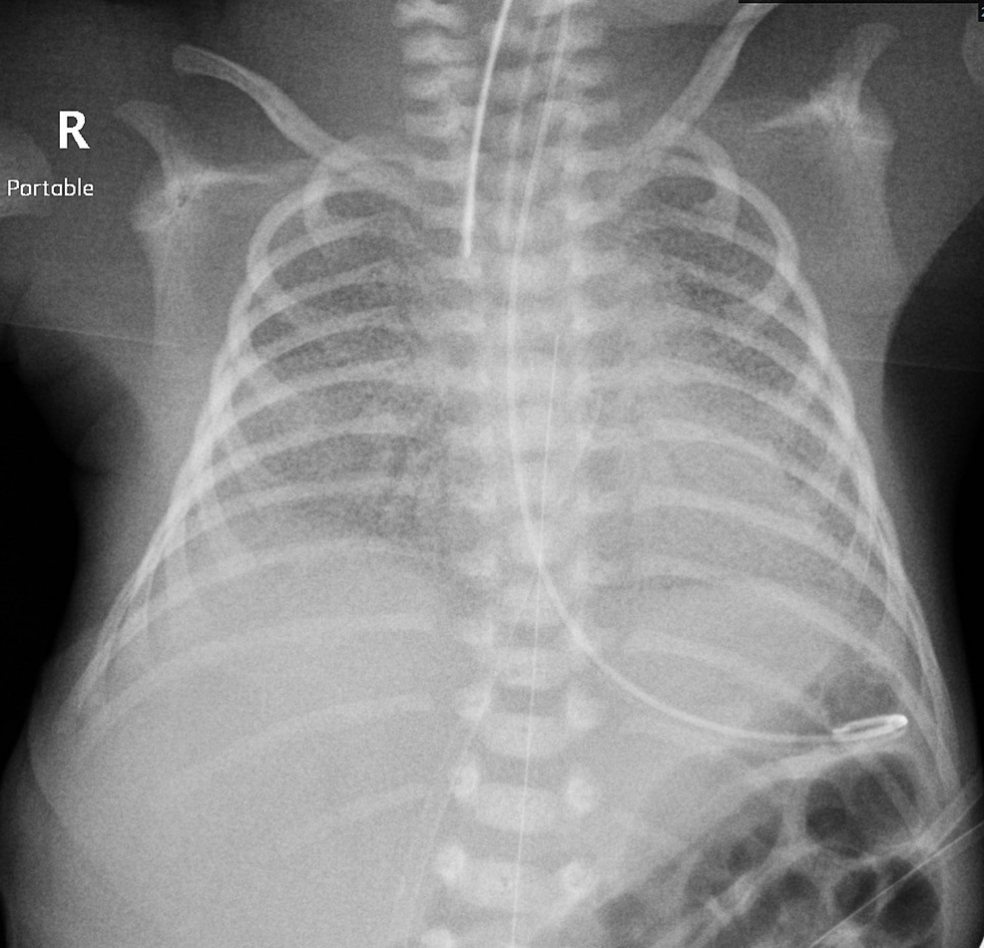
Chest radiography showed worsening respiratory distress syndrome but no pneumothorax.
